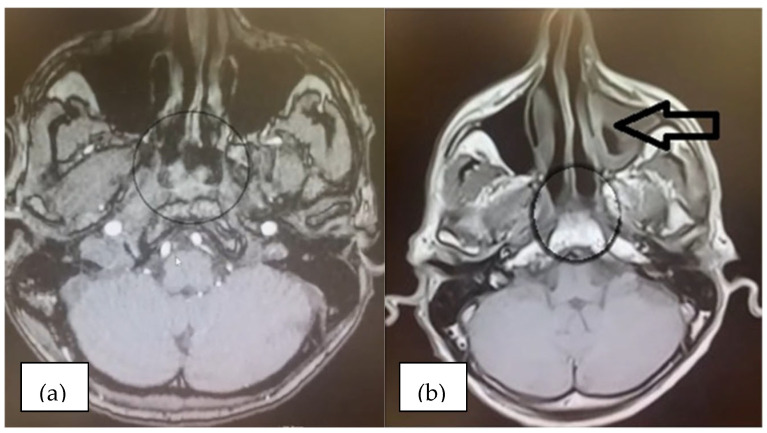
MRI examination of the cerebral regions: aspects before and after chemotherapy: (a) initial aspect of the nasopharyngeal tumor mass (circle); (b) visible tumor size reduction (circle) with subsequent inflammation of the left maxillary sinus mucosa (arrow).
